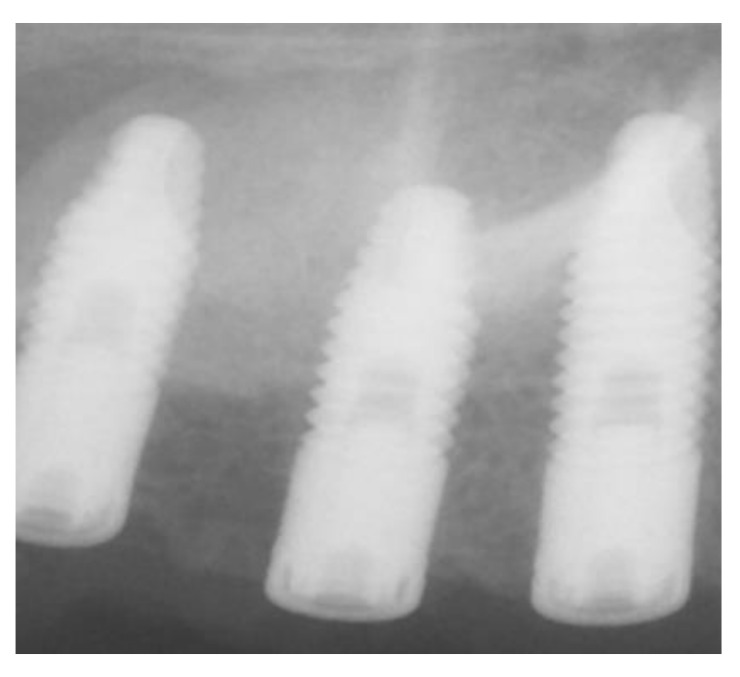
X-ray after installing the implants.
